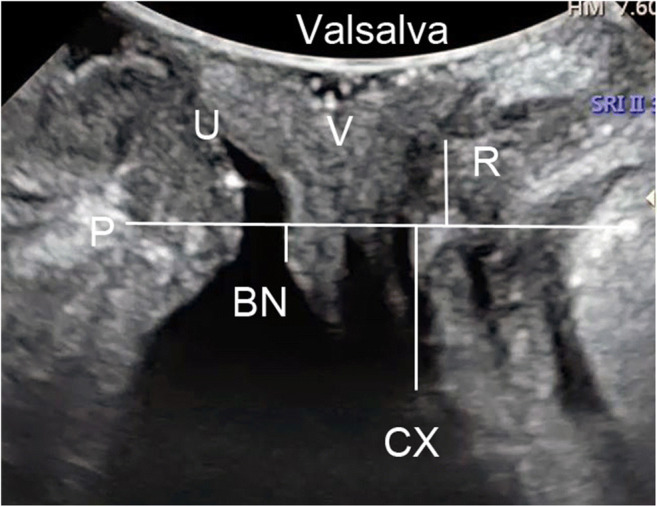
Transperineal ultrasound image in the mid-sagittal plane demonstrates the descent of the bladder, uterus and rectal ampulla during real-time leaking in the cough stress test. Measurements represent the maximally caudal organ position, which is the distance between the leading edge of the organs [bladder neck (BN), cervix (CX) and rectal ampulla (R)] and the horizon line at the level of the posterior inferior margin of the pubic symphysis (P). Measurements cranial to the reference line are positive; those caudal to the line are negative. P: pubic symphysis, BN: bladder neck, CX: cervix, U: urethra, V: vagina, R: rectal ampulla
